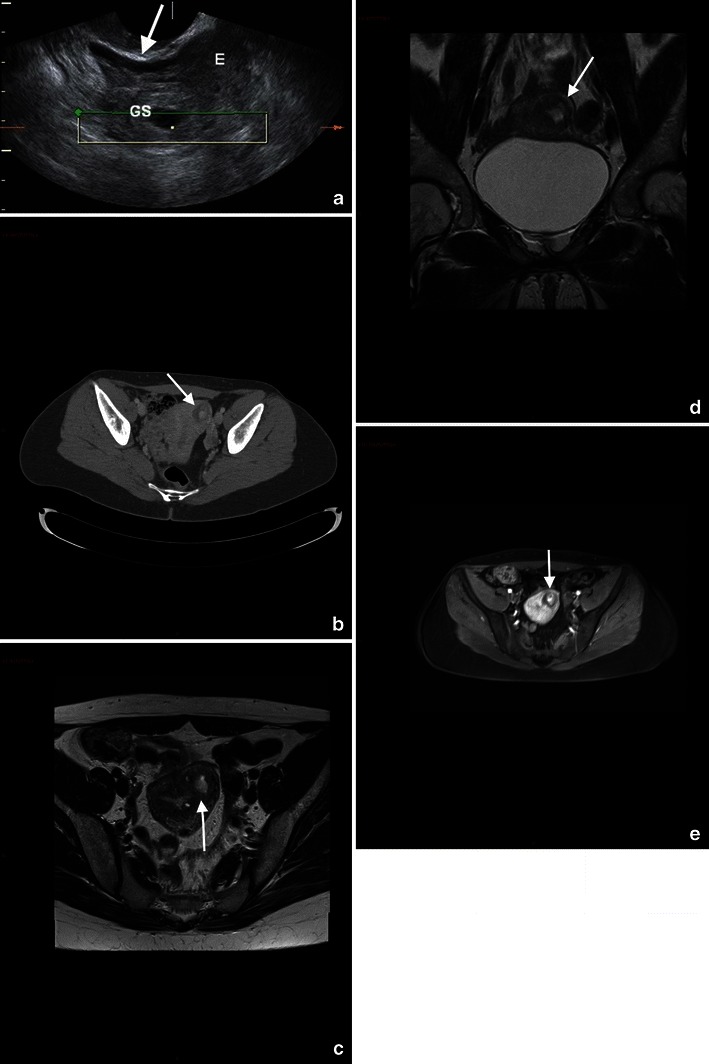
A 35-year-old woman presented with amenorrhea for 7 weeks, abdominal pain and vaginal bleeding; the B-HCG level was elevated. Transvaginal 3D sonogram in sagittal scan shows the interstitial portion of the tube (arrow) located between the gestational sac (GS) and endometrium (e). CT of the pelvis (b) shows a small lesion on the left side of the uterus. Axial (c) and coronal (d) T2-weighted images and axial contrast T1-weighted image (e) of the pelvis show a left gestational-like structure measuring 10 mm in diameter, surrounded by a thick wall according to interstitial pregnancy
